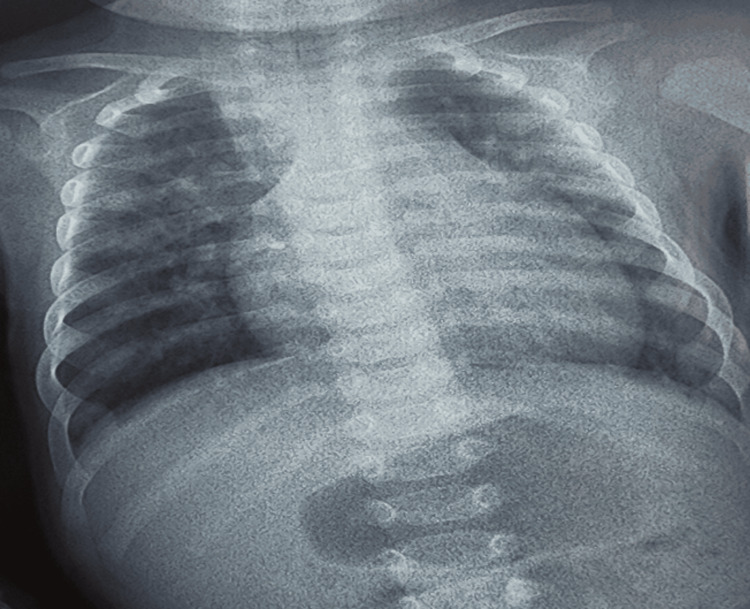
Chest X-ray showing cardiomegaly with pulmonary hypervascularisation
